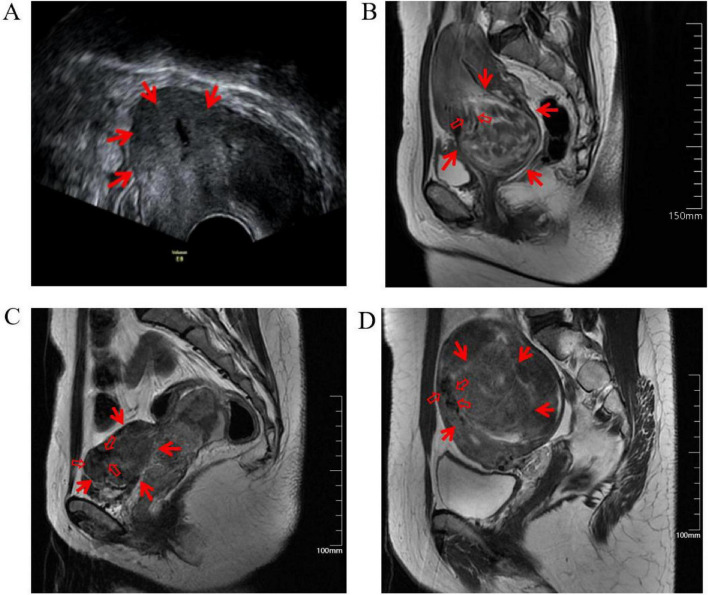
Preoperative imaging findings of ALM. (A) Preoperative ultrasound image: A space-occupying lesion measuring approximately 5.1 cm × 4.1 cm × 2.5 cm is seen in the right uterine wall, with tortuous and dilated peritumoral veins surrounding the lesion in an “worm-like” pattern. (B) Type 0 submucosal angioleiomyoma on pelvic MRI T2WI: A mass measuring approximately 6.0 cm × 5.8 cm × 5.0 cm is observed in the submucosa of the anterior uterine wall, partially protruding into the vagina, with abundant cord-like vascular signals within the tumor; the solid arrow indicates the main tumor body, and the open arrow indicates abnormal vessels. (C) Broad ligament angioleiomyoma on pelvic MRI T2WI: Multiple fused nodular lesions measuring approximately 9.2 cm × 4.7 cm × 4.2 cm are seen adjacent to the left uterine horn, accompanied by local necrosis, with coarse vascular signals visible at the lesion margin; the solid arrow indicates the main tumor body, and the open arrow indicates abnormal vessels. (D) Intramural angioleiomyoma on pelvic MRI T2WI: A round-like nodule measuring approximately 7.8 cm × 7.9 cm × 6.4 cm is seen in the posterior wall of the uterine fundus, with a cluster of coarse and tortuous vessels at the margin; the solid arrow indicates the main tumor body, and the open arrow indicates abnormal vessels.
